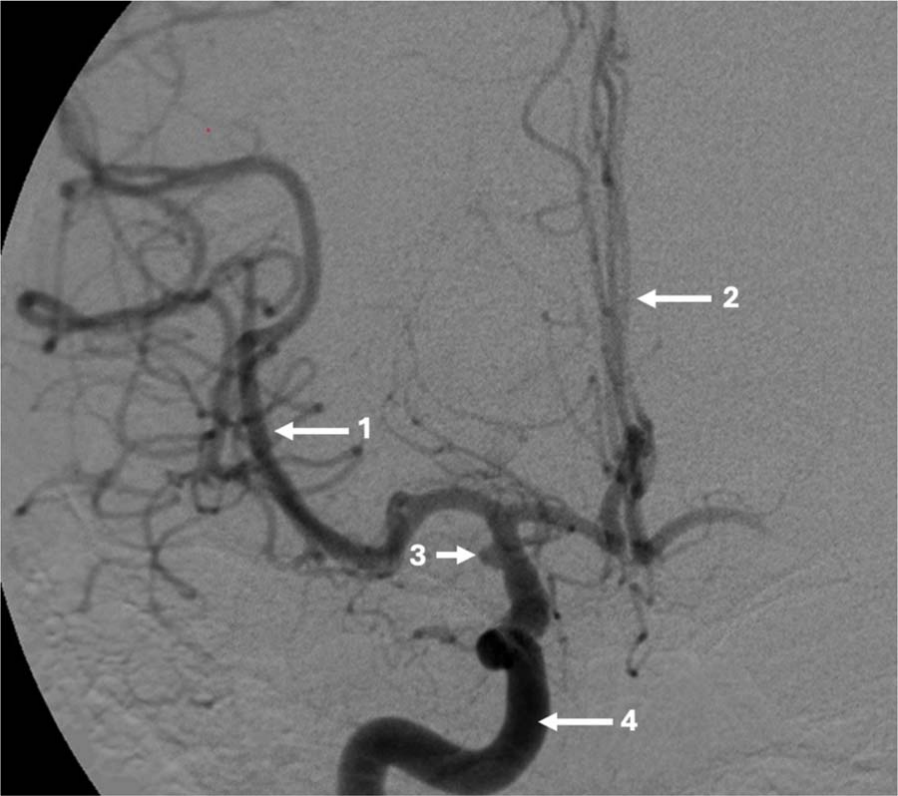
Pre-operative CT angiogram demonstrates normal appearances of the (1) MCA, (2) ACA with, (3) laterally projecting terminal carotid sidewall aneurysm. (4) R ICA.
